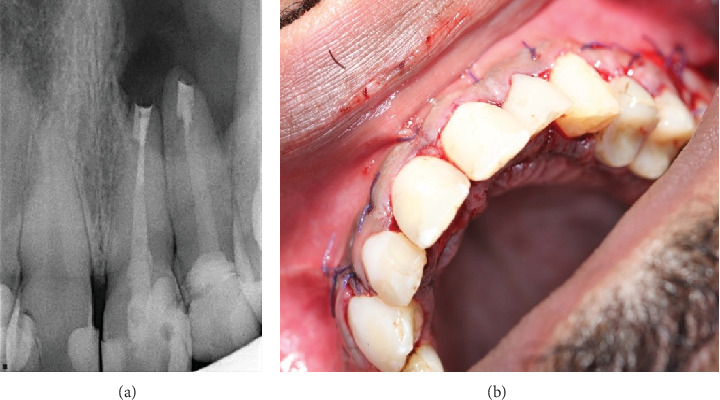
(a, b) Immediate postoperative clinical PA X-ray after placement of retrofill material and postoperative image after flap repositioning and suturing.
